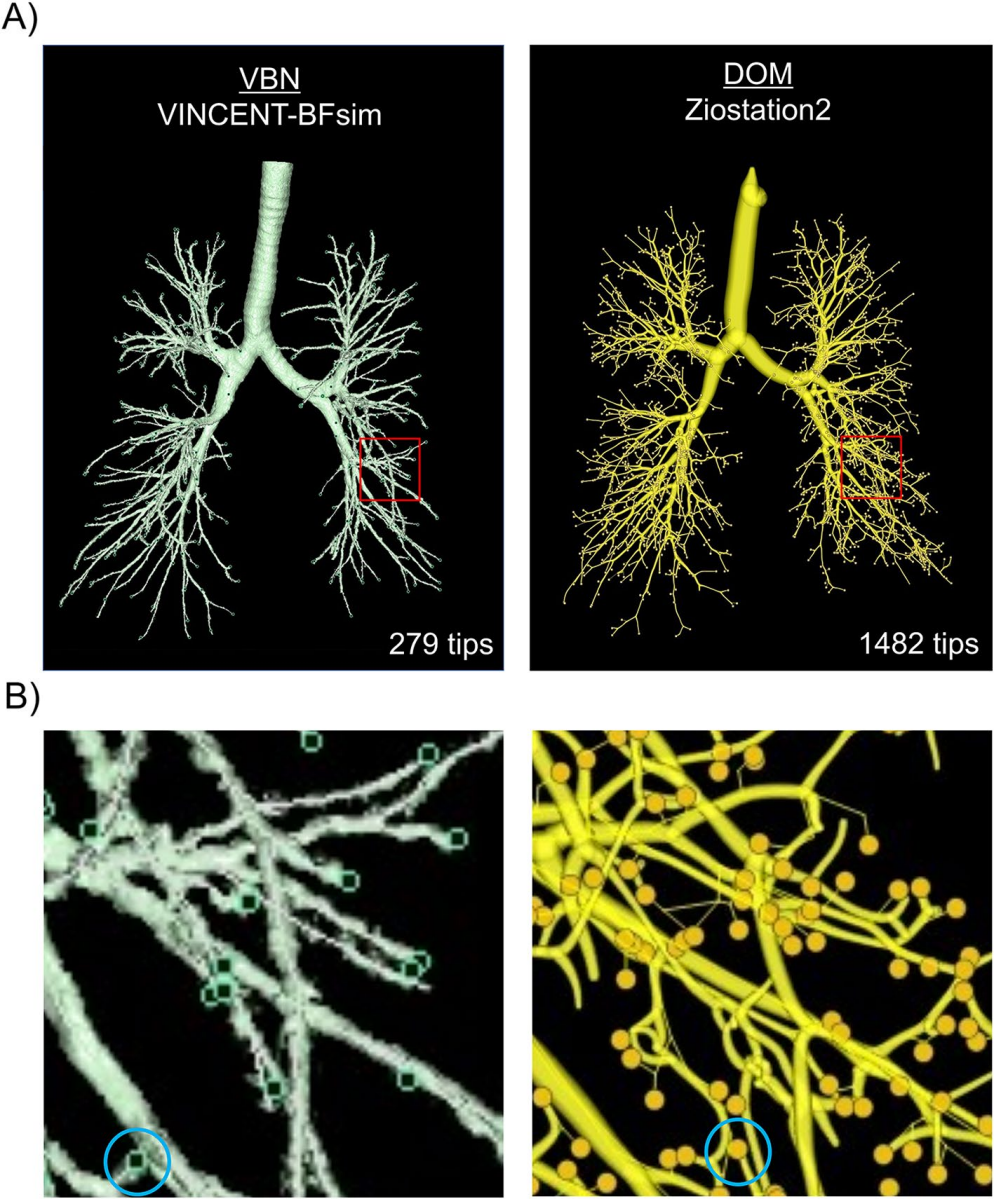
Bronchial trees were reconstructed by automatic CT analysis using virtual bronchoscopic navigation software and by manual CT analysis using the direct oblique method (DOM). (A) Images of the entire bronchial trees. The virtual bronchoscopic navigation software, VINCENT-BFsim, identified 279 bronchial tree tips in the whole lung, while the DOM on Ziosation2 identified 1482 tips. The areas indicated by red squares are magnified in (B). (B) Magnified images of the bronchial trees. The tips of the bronchial trees are shown as round dots. The blue circles indicate examples of tips.
